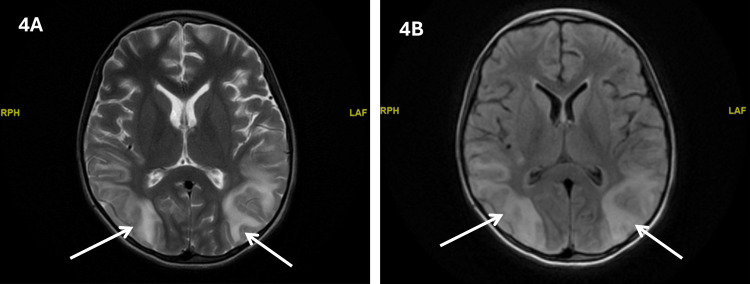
Brain MRI suggestive of posterior reversable encephalopathy. (A) Axial T2-weighted brain MRI showing bilateral posterior parieto-occipital cortico-subcortical hyperintense areas (white arrows), in keeping with vasogenic edema/posterior reversible encephalopathy (PRES) pattern. (B) Axial FLAIR (fluid-attenuated inversion recovery) image demonstrating symmetric posterior cortical-subcortical hyperintensity in the parieto-occipital regions (white arrows), compatible with PRES.
